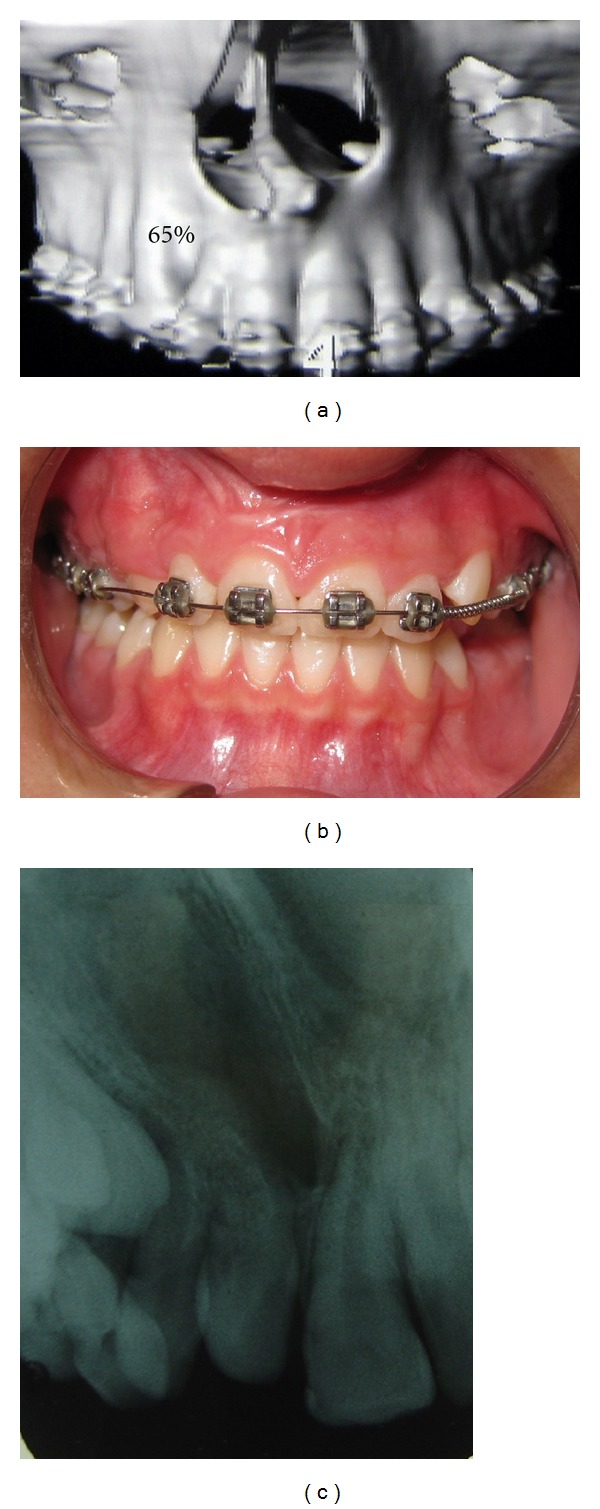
Patient with grade II. (a) CT scan view; (b) occlusal view; (c) dental X-ray.
